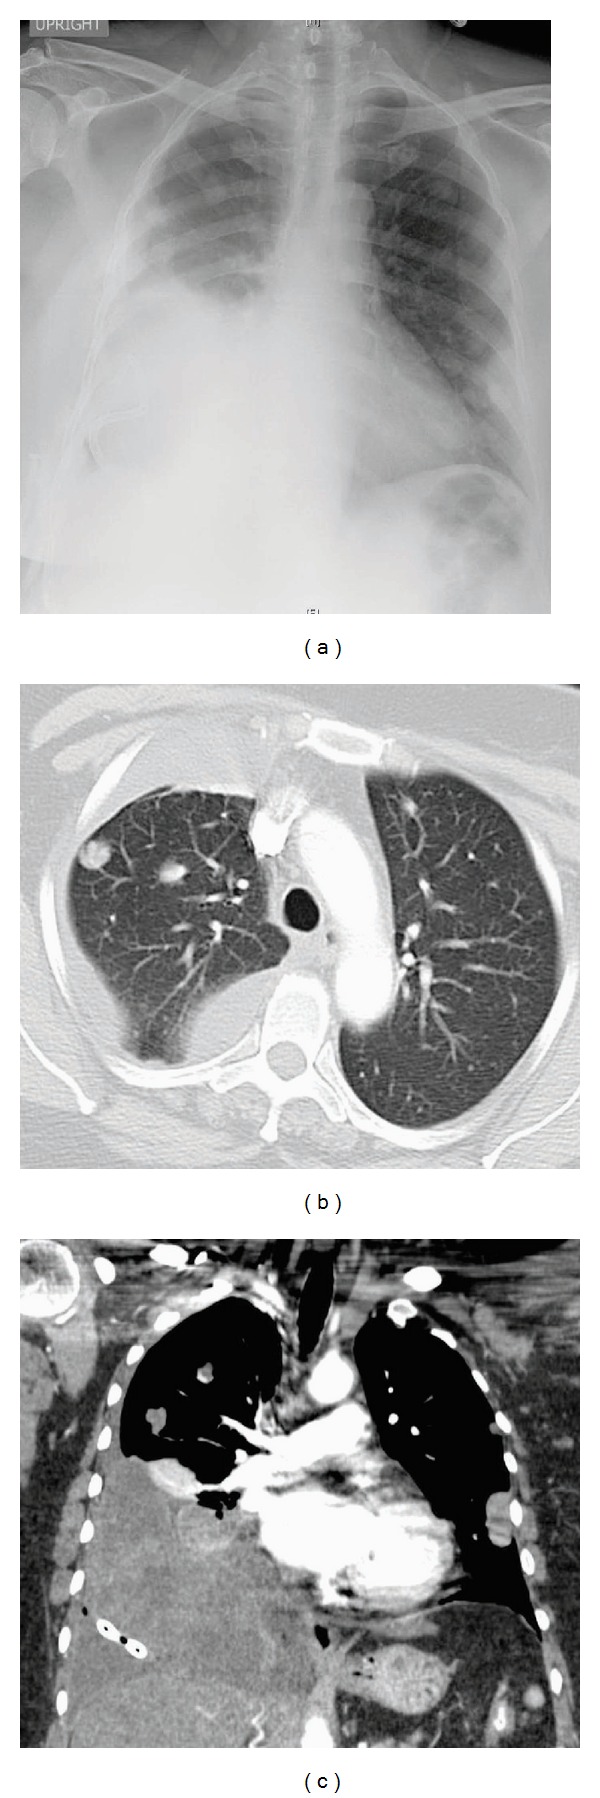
(a) CXR showing residual lower lobe collection of fluid after insertion of chest tube. (b) Transverse section of thorax by CT showing restoration of lung parenchyma in the upper lobe of the right lung. (c) Coronal section of thorax by CT showing a significant collection of fluid in the middle and lower right lobes of the lung.
